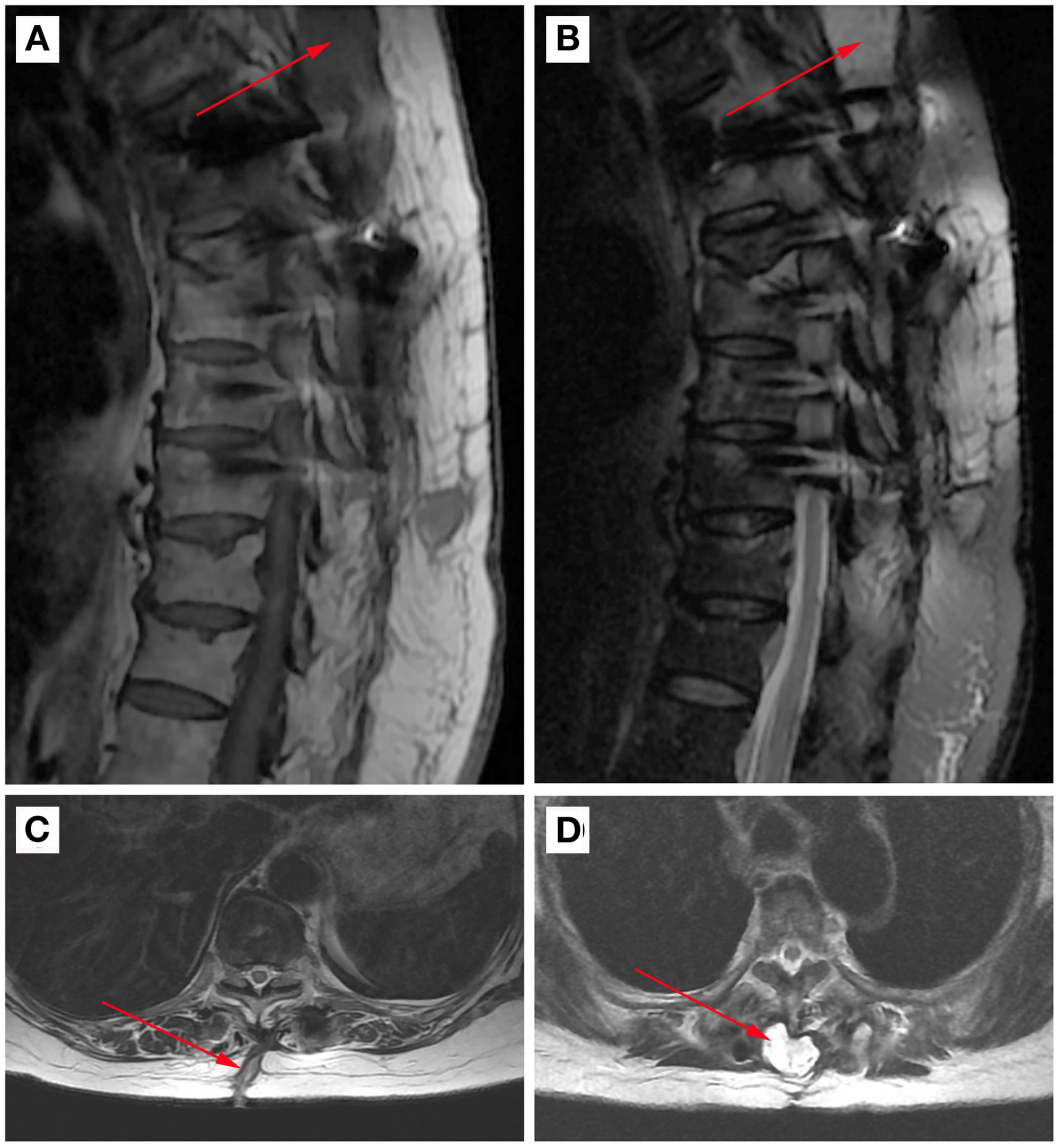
A typical case of post-operative wound infection of spinal tuberculosis. The arrow marks the location of inflammatory pus. (A) Post-operative MRI image of the sagittal position T1 sequence. (B) Post-operative MRI image of the sagittal position T2 sequence. (C) Post-operative MRI image of the cross-section T1 sequence. (D) Post-operative MRI image of the cross-section T2 sequence.
